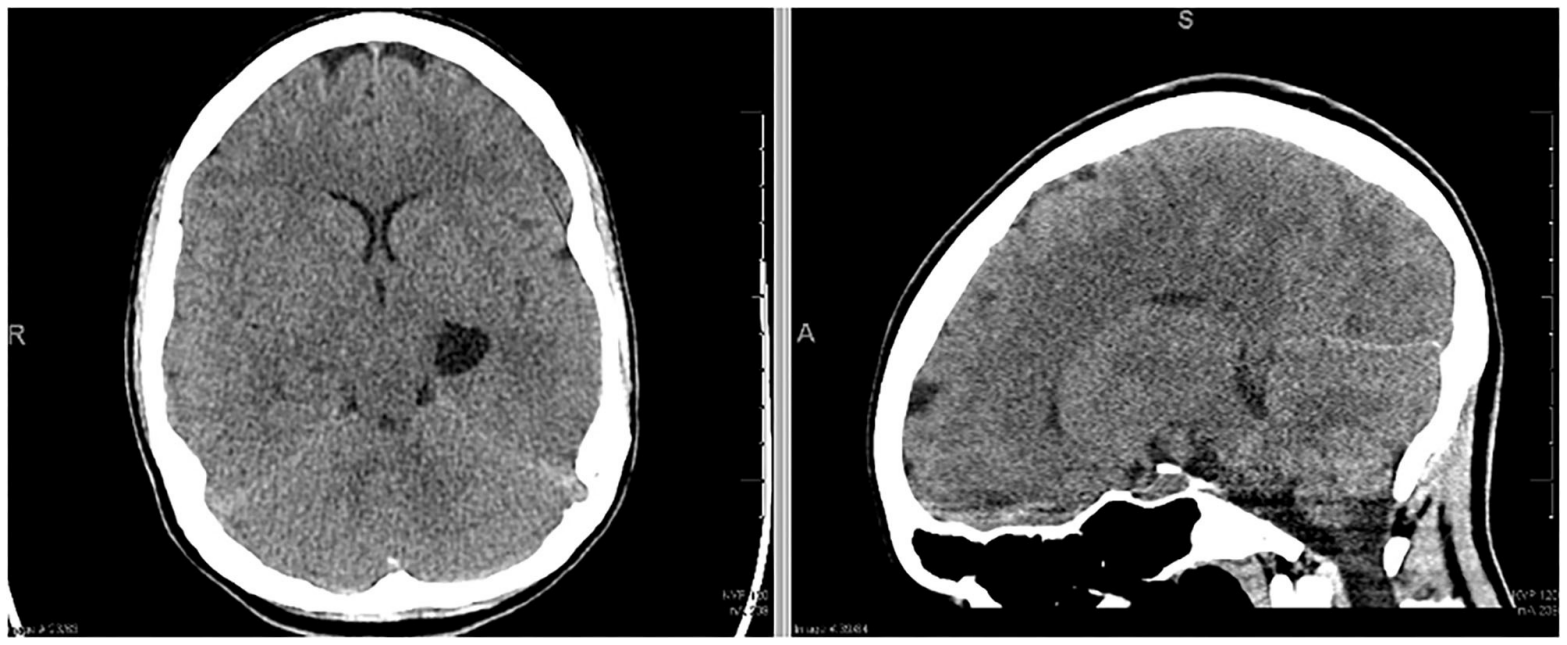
Initial non-contrast CT of the head demonstrating left temporal cyst.
